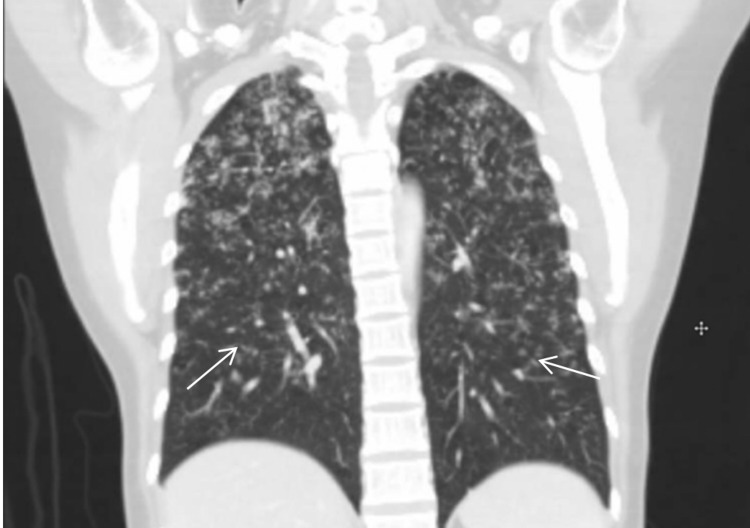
Coronal chest CT imaging showing diffuse tree-in-bud appearance with micro-nodularity involving upper and lower lobes. CT: computed tomography
